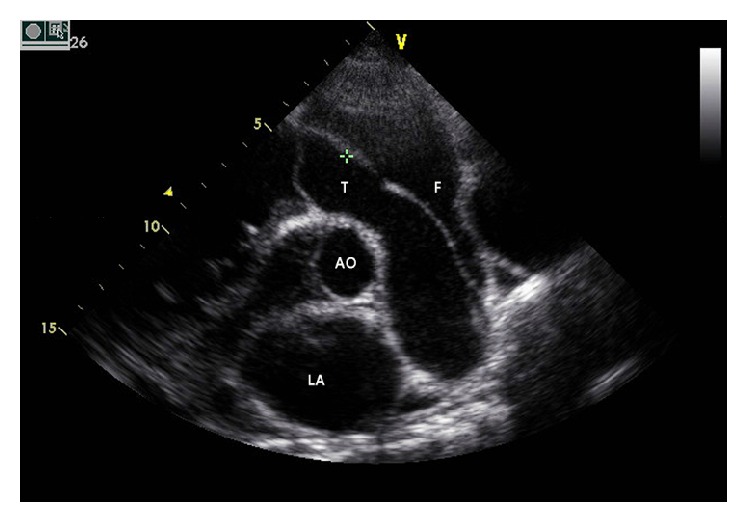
Echocardiogram. Two-dimensional transthoracic echocardiography in short-axis view of the heart base showing a greatly dilated pulmonary trunk with the dissection membrane (+). AO, aorta; LA, left atrium; T, true lumen; F, false lumen.
